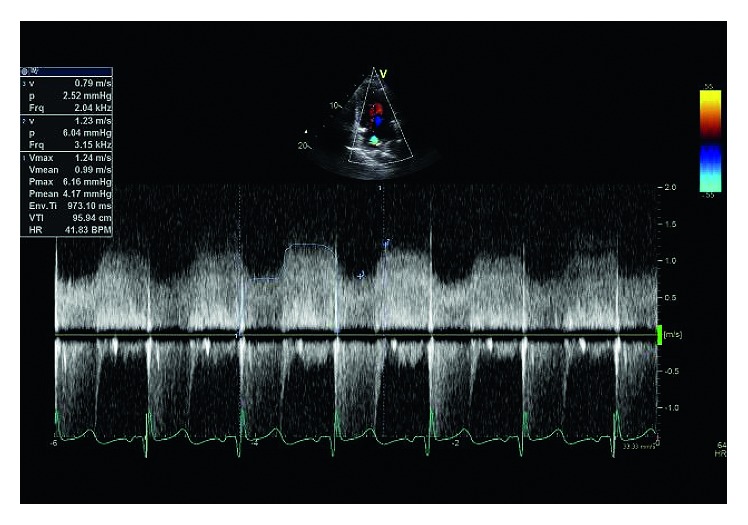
Continuous wave Doppler signal across the opening of the cor triatriatum membrane showing mildly accelerated flow throughout the cardiac cycle. The peak diastolic gradient was measured at 6 mmHg.
